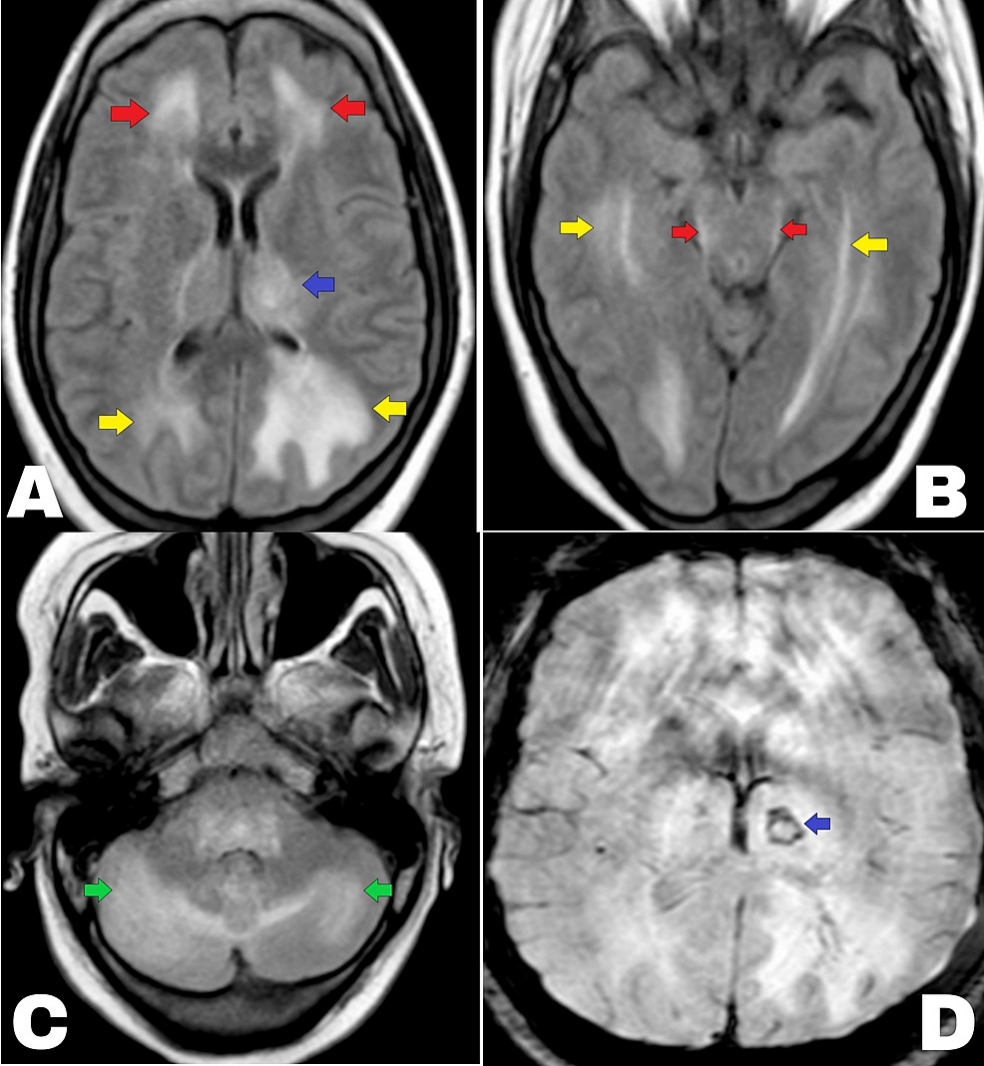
Magnetic resonance imaging (fluid-attenuated inversion recovery imaging) demonstrating hyperintensities in bilateral cerebral [frontal lobes (red arrow) and left thalamus (blue arrow) and occipital lobes (yellow arrow) in A], midbrain (red arrow) and temporal lobes (yellow arrow) in B, and cerebellar hemispheres (green arrow in C). Susceptibility-weighted imaging (D) suggested thalamic bleed (blue arrow).
